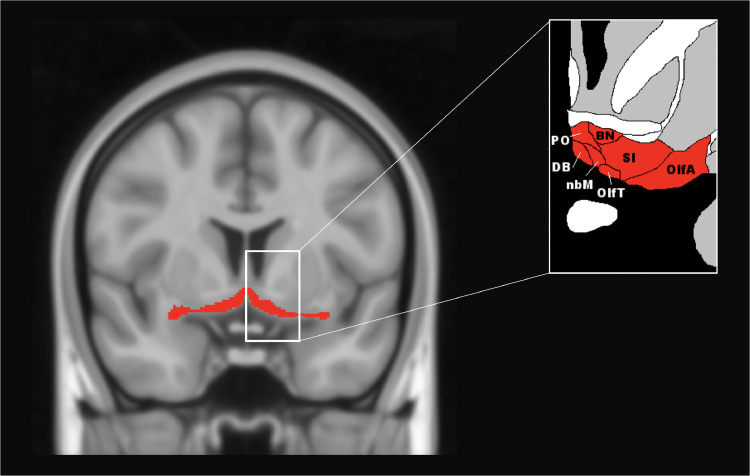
Representative figure of the basal forebrain and its anatomy, based on Ding et al. (2016). The basal forebrain segmentation is mapped on to the T1-weighted ICBM152 2009c nonlinear symmetric - 1 × 1 × 1 mm template (McConnell Brain Imaging Centre, Montreal Neurological Institute, McGill University). PO = preoptic area, BN = bed nucleus, DB = nucleus of the diagonal band, nbM = basal nucleus of Meynert, OlfA = olfactory area, OlfT = olfactory tract, SI = substantia innominata. The coronal slice corresponds to MNI *y* = 131.
